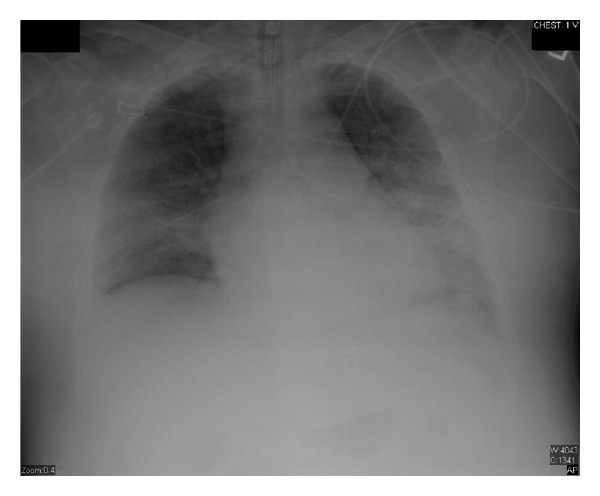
Chest X-ray day 18 after chemotherapy (ANC was 1100 cell/*μ*L).
